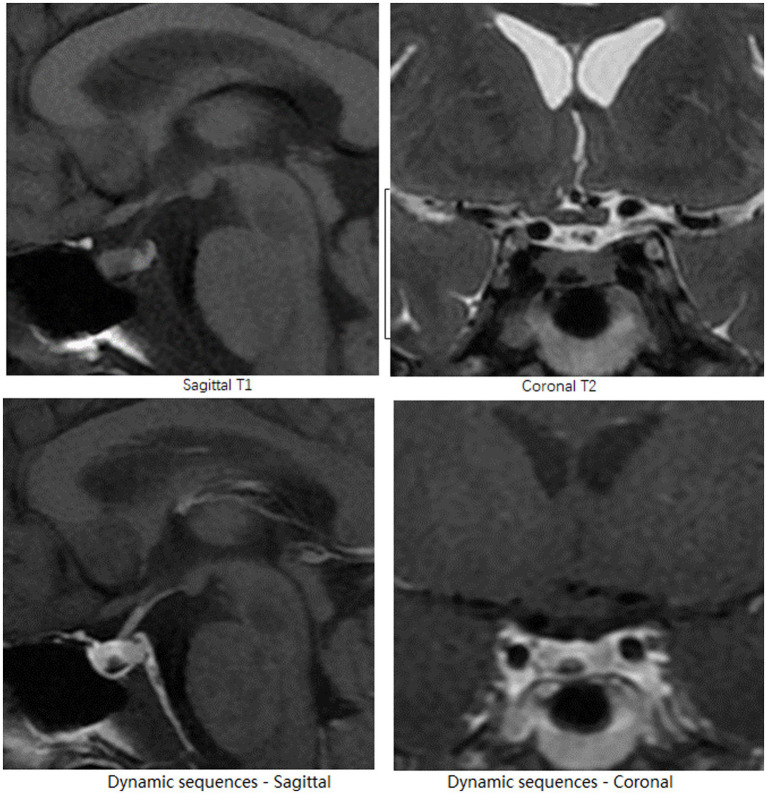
Head MRI. On the right side of the pituitary gland, the pituitary microadenoma, appears as a nodular lesion with a hypointense signal on sagittal T1-and coronal T2-weighted pre-enhanced MRI images, and demonstrates delayed hyperenhancement on dynamic sequences.
